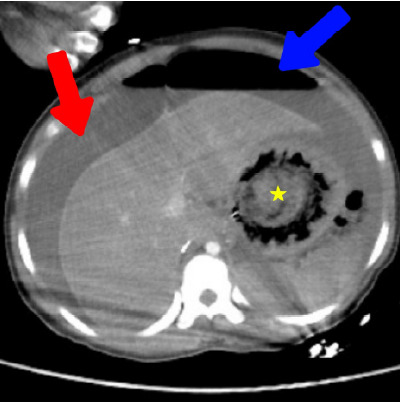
Abdominal computed tomography (axial plane) image at the level of the liver and stomach. Free intraperitoneal fluid (red arrow) and pneumoperitoneum (blue arrow) are present. A large intragastric mass consistent with a bezoar is seen (yellow star).
